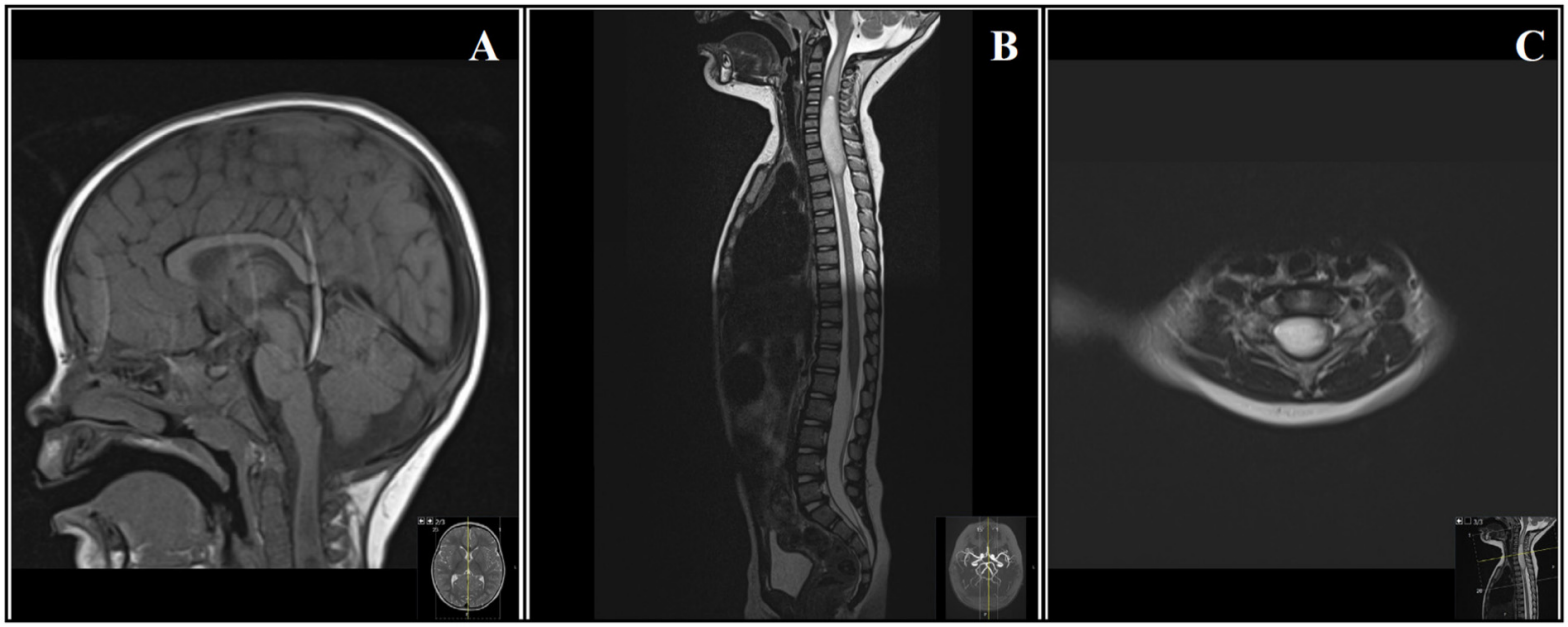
Magnetic Resonance Imaging. (A) Sagittal T1-weighted imaging of the brain, with the upper part of the cervical cord visible, showing a mass lesion. (B) Sagittal T2-weighted imaging of the spinal cord, showing an intramedullary mass in the cervicothoracic spinal cord (C5-Th3). (C) Axial T2-weighted imaging of the spinal cord, showing the compressive effect of the mass lesion, predominantly on the right C6 nerve root.
